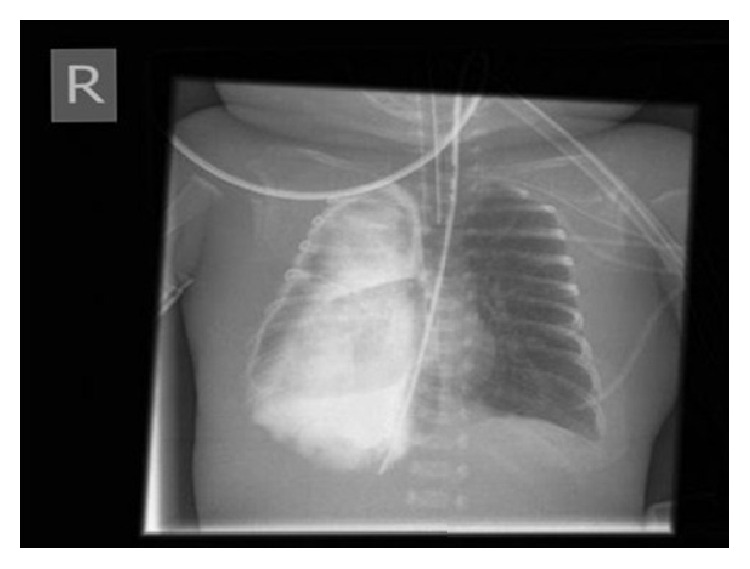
Chest radiograph after contrast agent application showing massive effusion in the pleural cavity and abnormal right-sided position of the nasogastric tube.
